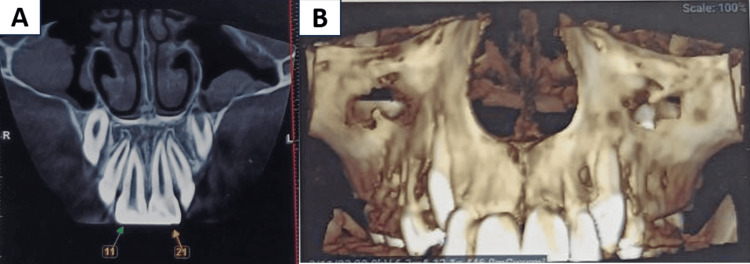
CBCT examination in images A and B revealing no fractures in the region of the maxillary anterior teeth CBCT: cone beam computed tomography
